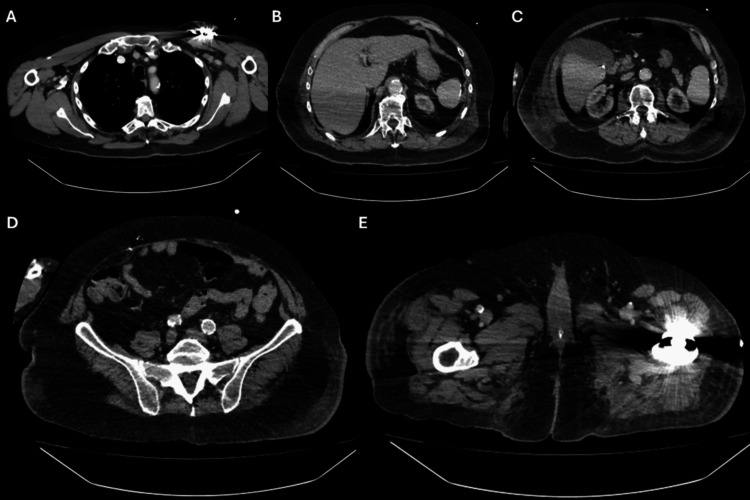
Axial contrast-enhanced computed tomography (CT) images. (A) Chest at the level of the thoracic inlet, with artefact from a right-sided pacemaker overlying the chest wall. (B) Upper abdomen. (C) Abdomen at the level of the renal hila. (D) Lower abdomen and pelvis. (E) Pelvis demonstrating bilateral hip prostheses with associated streak artefacts.
